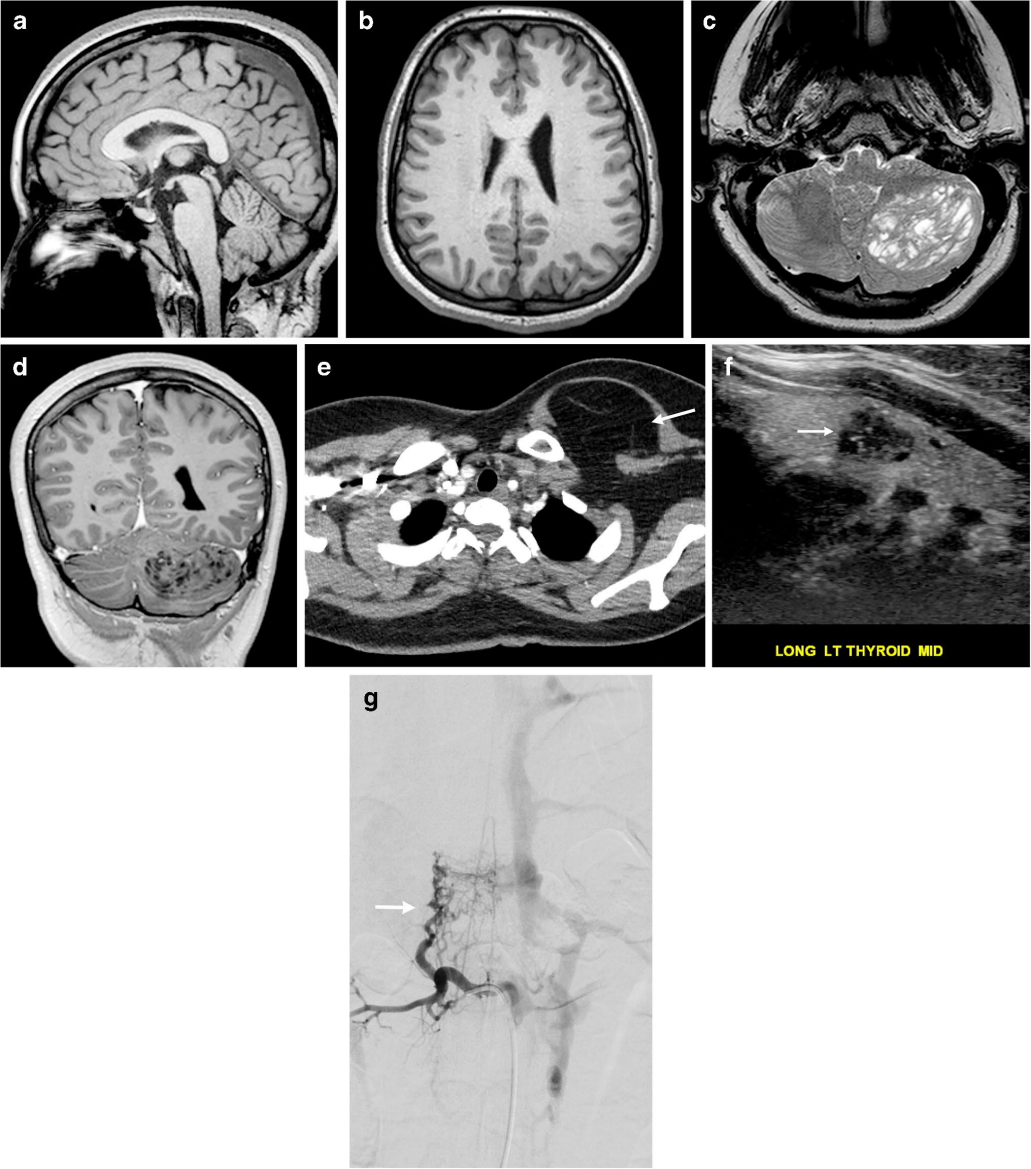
Cowden syndrome in a 14-year-old girl with a genetically confirmed *PTEN* gene mutation with multiple systemic anomalies. a, b Sagittal and axial spoiled gradient recalled echo images show a mildly enlarged corpus callosum and excess white matter. c, d Axial T2-weighted and coronal contrast-enhanced T1-weighted images show a striated T2 hyperintense nonenhancing lesion in the left cerebellar hemisphere, which was consistent with a dysplastic gangliocytoma—Lhermitte-Duclos disease—after resection. e An axial contrast-enhanced chest computed tomography scan shows a large left chest wall lipoma (*arrow*). f A longitudinal ultrasound shows a left thyroid nodule (*arrow*) with irregular borders and punctate echogenic foci that was compatible with a papillary thyroid cancer after surgical resection. g A conventional spinal angiogram in the anteroposterior projection with the patient supine with injection from the right L1 lumbar artery shows a spinal arteriovenous malformation (*arrow*)
